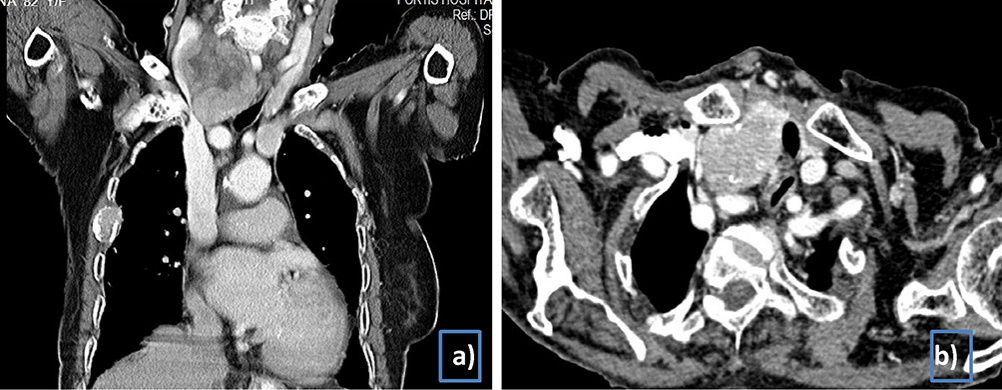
(a, b) Pre-operative contrast-enhanced CT scan of the neck and chest. A large enhancing mass lesion with central necrosis in the right lobe of the thyroid causing compression and deviation of the trachea to the left side.
